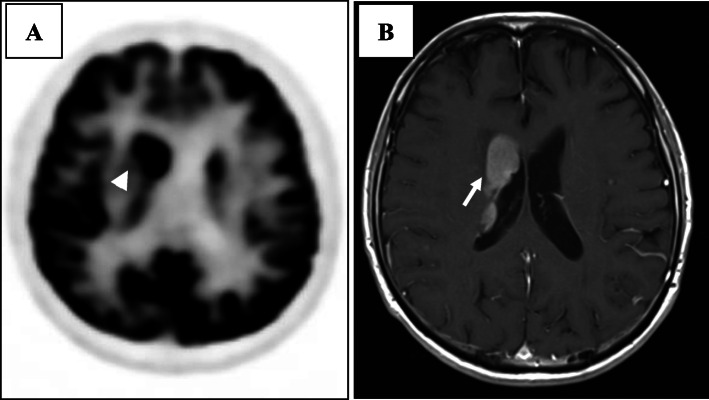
Head imaging at the time of central nervous system spread. (A) PET-CT showed abnormal accumulation in the right frontal lobe (arrow head). (B) Contrast-enhanced MRI (T1) showed an enhancing effect at the same site (arrow)
